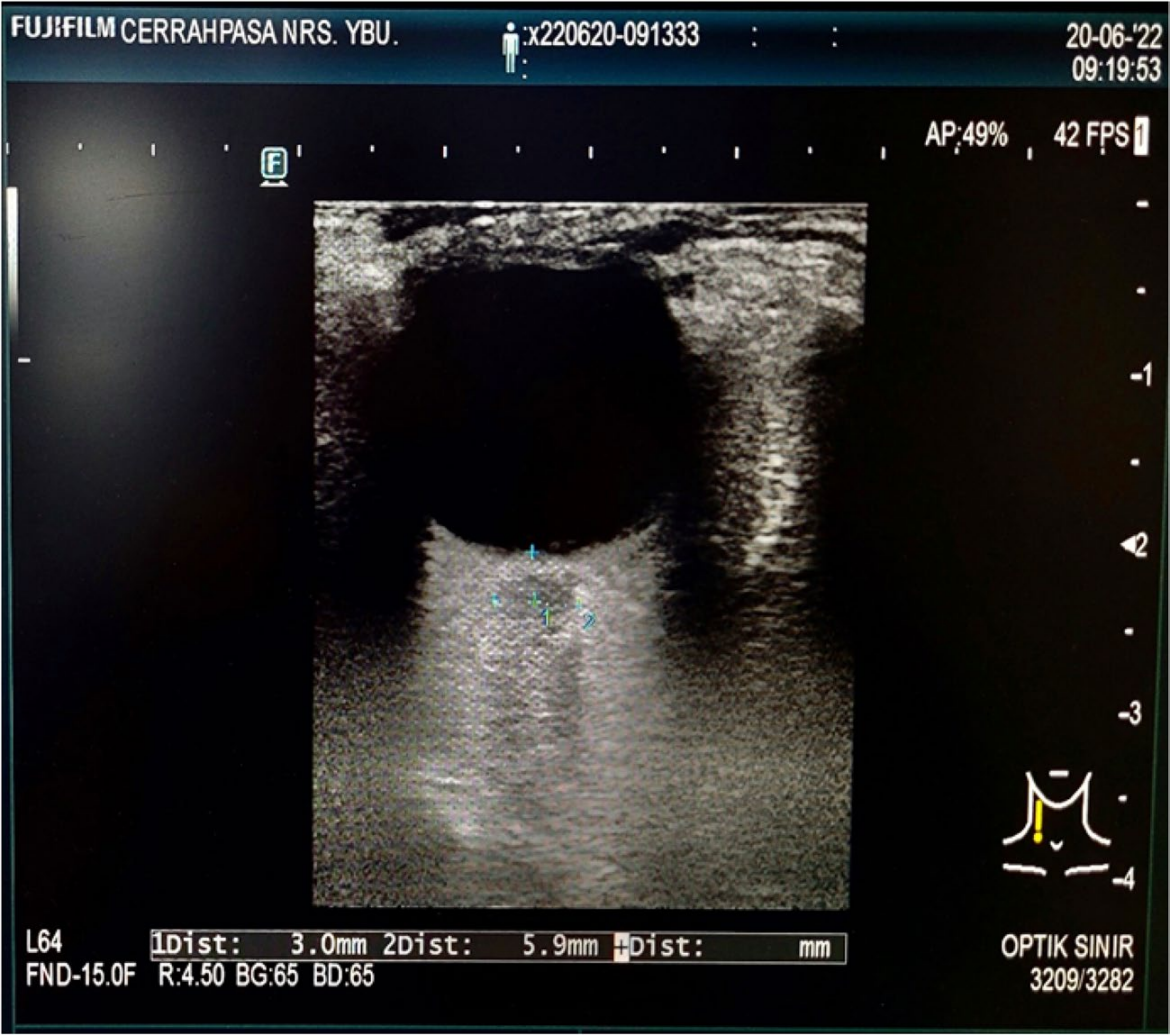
Ultrasonography guided OSND measurement
